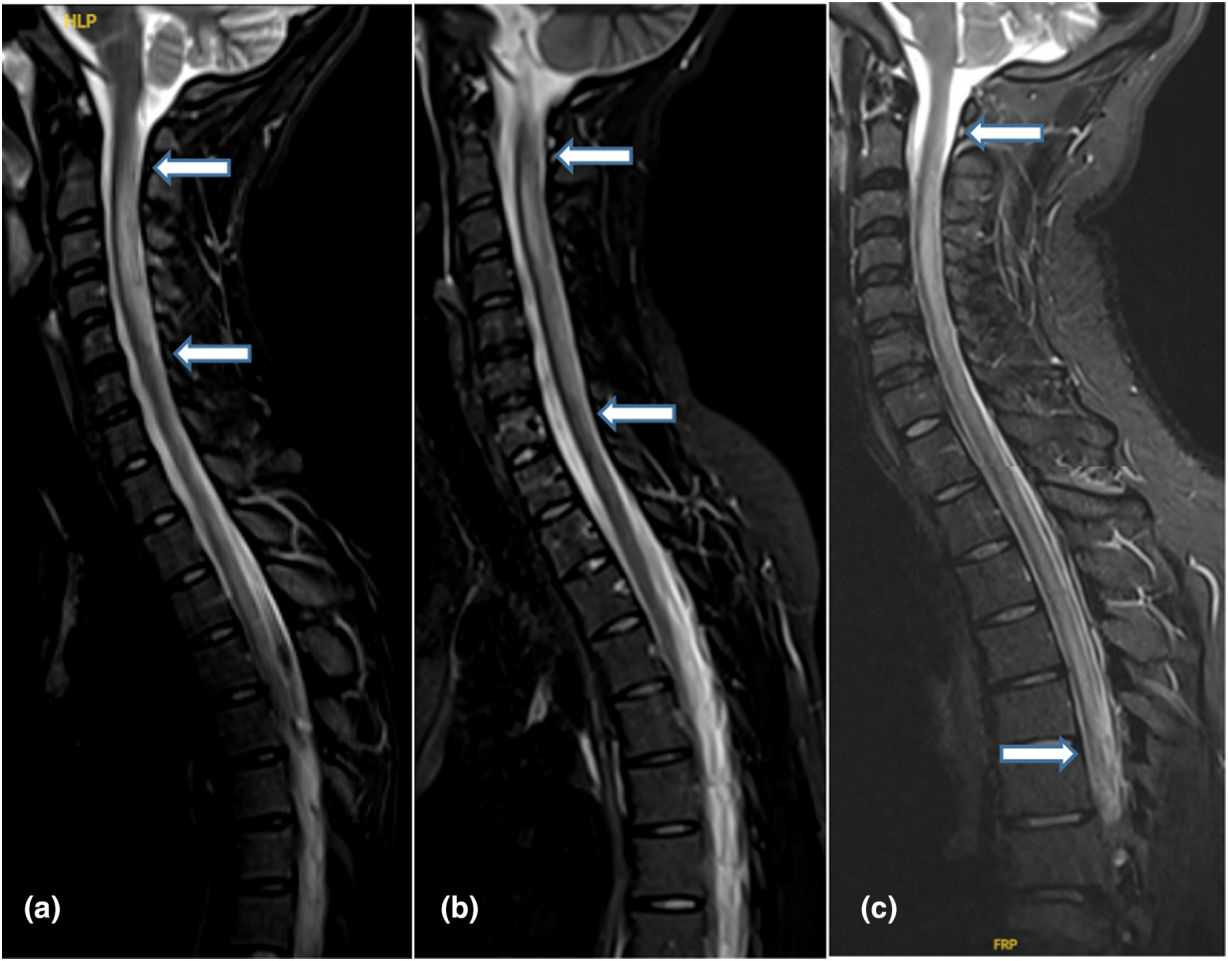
Myelitis extension according to N_2_O consumption. (a) Spinal magnetic resonance imaging (MRI; sagittal section) of a 25‐year‐old patient presenting a C2–C5 myelitis. Median weekly N_2_O consumption = 4 cylinders. (b) Spinal MRI (sagittal section) of a 22‐year‐old patient presenting a C2–C7 myelitis. Median weekly N_2_O consumption = 7 cylinders. (c) Spinal MRI (sagittal section) of a 25‐year‐old patient presenting a C2–T6 myelitis. Median weekly N_2_O consumption = 21 cylinders.
